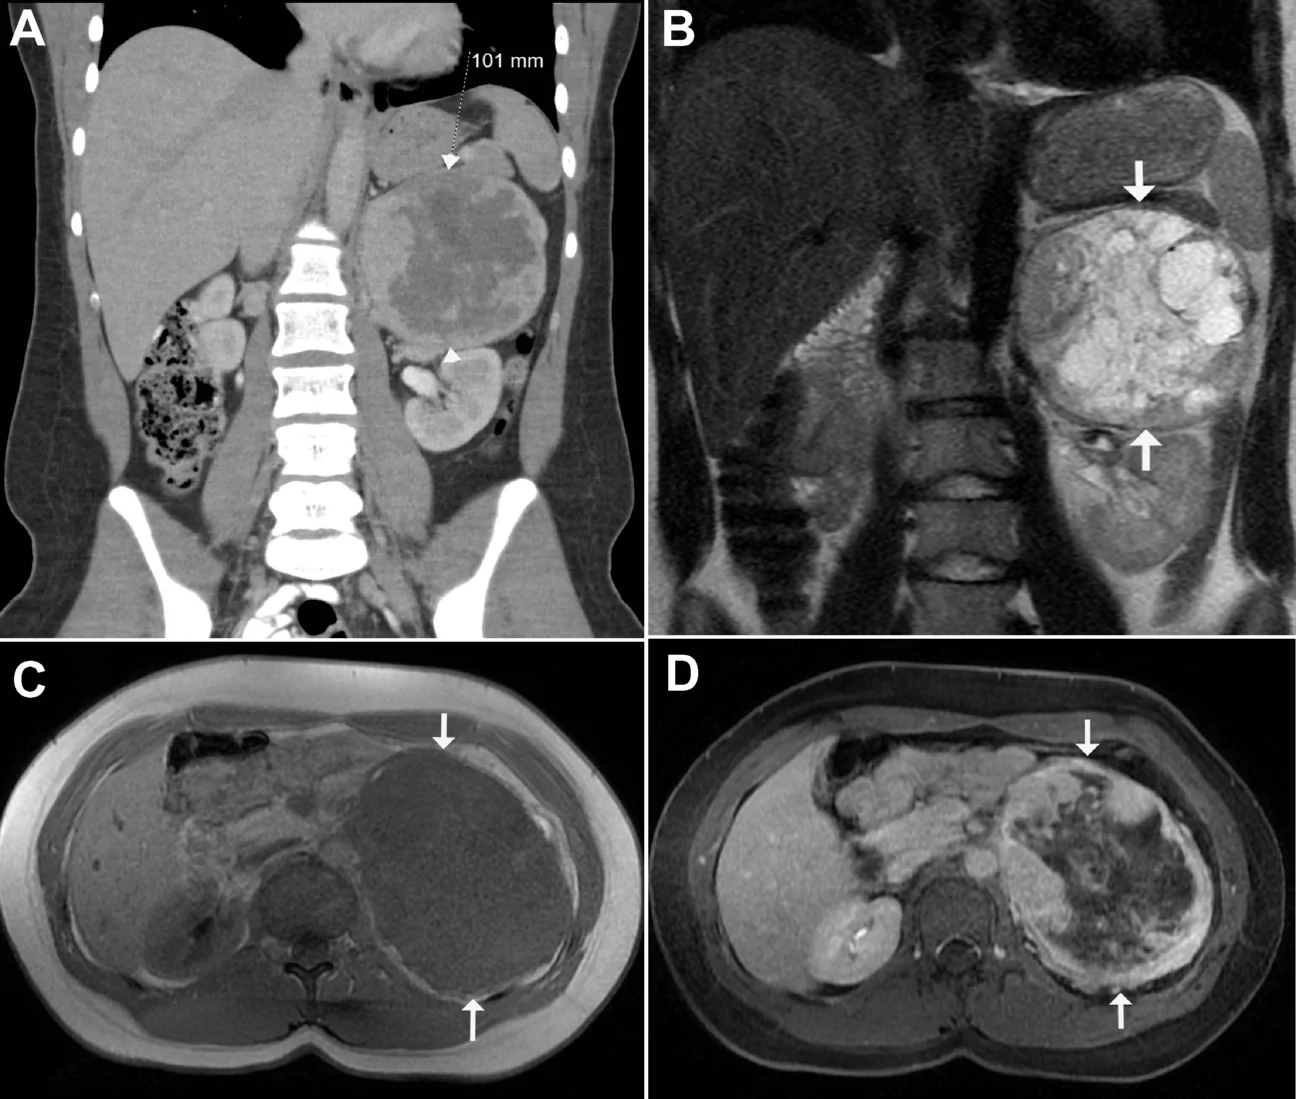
Pheochromocytoma in a 17-year-old female with shortness of breath and palpitation but normotensive. a Contrast-enhanced abdominal CT shows a large 10-cm left suprarenal mass with peripheral nodular enhancement and central low attenuating solid tissue (*arrowheads*). b  Coronal T2-weighted and (c) axial T1-weighted fat-suppressed pre-contrast and (d) post-contrast images show evidence of internal cystic components that are a frequent imaging feature of pheochromocytoma (*arrows* indicate mass margins)
